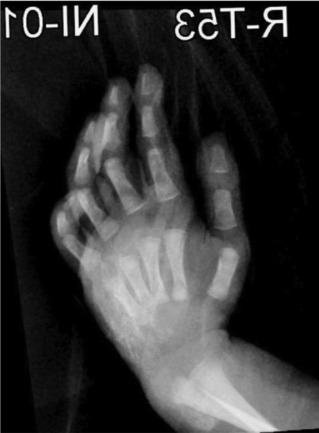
X-ray of the Right hand with flexion.
